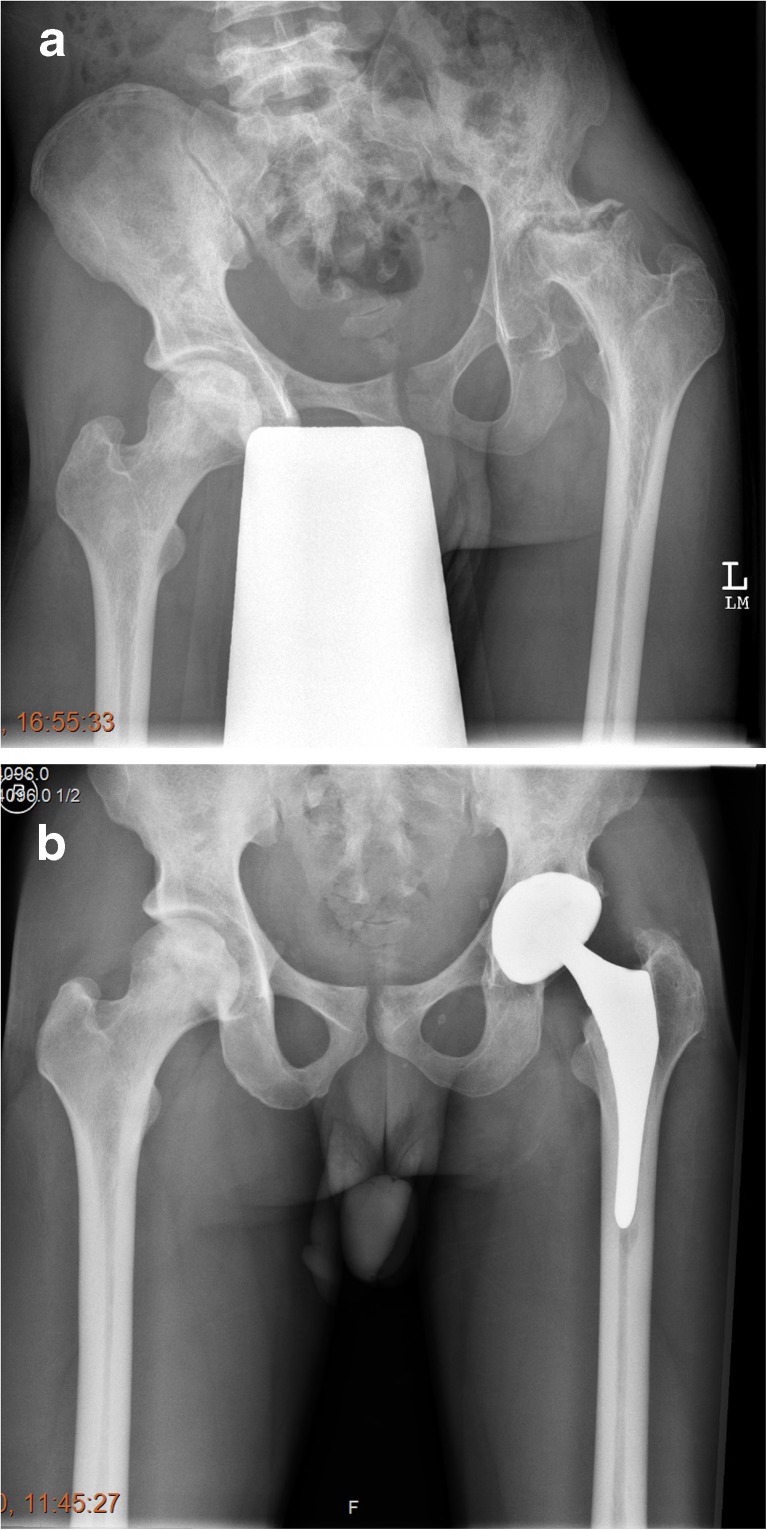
a Steinberg Stage VI Osteonecrosis left hip with severe femoral canal Stenosis and stage III right hip. b Radiographs 8 years postoperatively showing well-integrated Stryker ABG uncemented components
